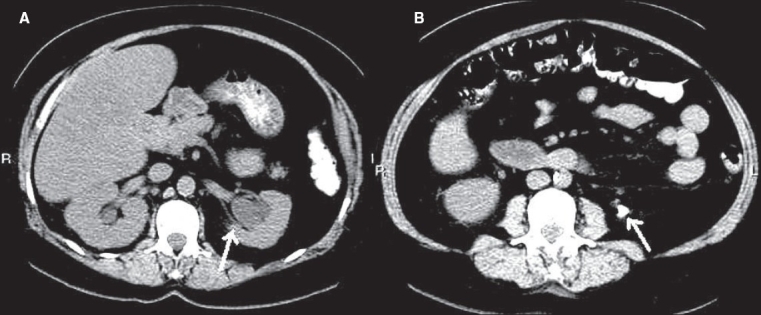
Ureteric calculus. Plain CT scan shows left hypdronephrosis (arrow in A) due to a 6 mm calculus (arrow in B). Note the absence of the ‘soft tissue rim’ sign
